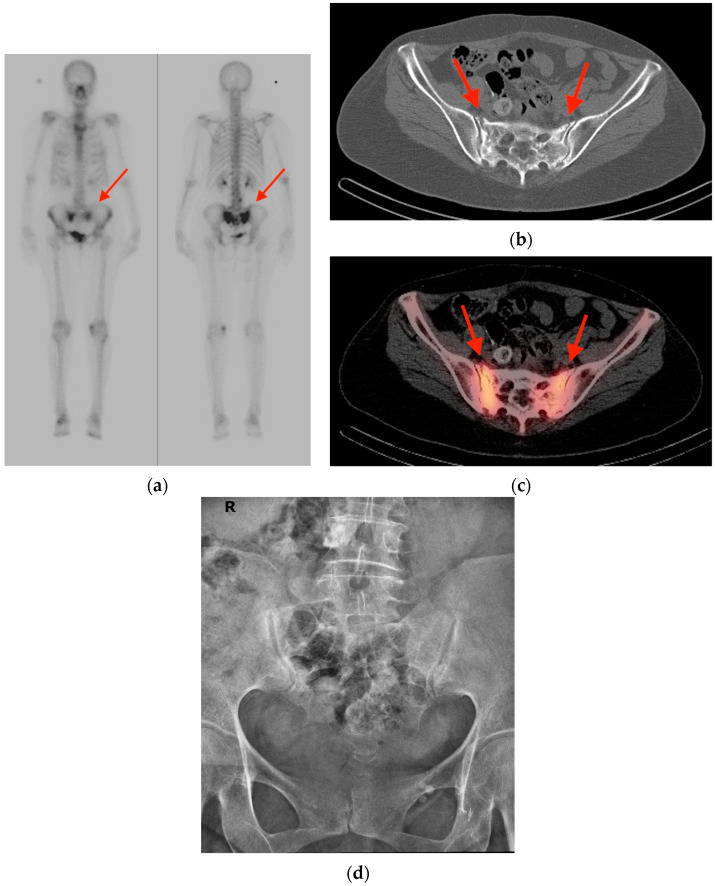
(a) Nuclear medicine bone scintigraphy scan obtained in a 62-year-old woman with colon adenocarcinoma and complaints of lower back pain shows a classic “H-sign” of focal uptake in the region of the sacrum (red arrows). (b) CT scan of the same patient shows cortical irregularities along the anterior aspect of the sacrum bilaterally (red arrows), consistent with fracture. (c) Fused PET/CT imaging reveals focal radiotracer uptake in the region of the fractures (red arrows). (d) Radiograph obtained in the course of work-up revealed no obvious abnormalities (R = right side of patient). CT = computed tomography. PET/CT = positron emission tomography/computed tomography.
